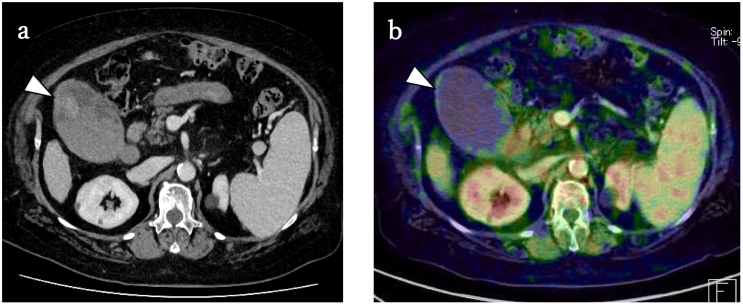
Contrast-enhanced computed tomography (CE-CT) at a follow-up visit 3 months after transcatheter arterial chemoembolization and radiofrequency ablation. (A) Abdominal CE-CT showed an irregularly shaped mass 22 mm in size at the fundus of the gallbladder (arrowhead). (B) Positron emission tomography-CT showed no accumulation in the gallbladder (arrowhead).
